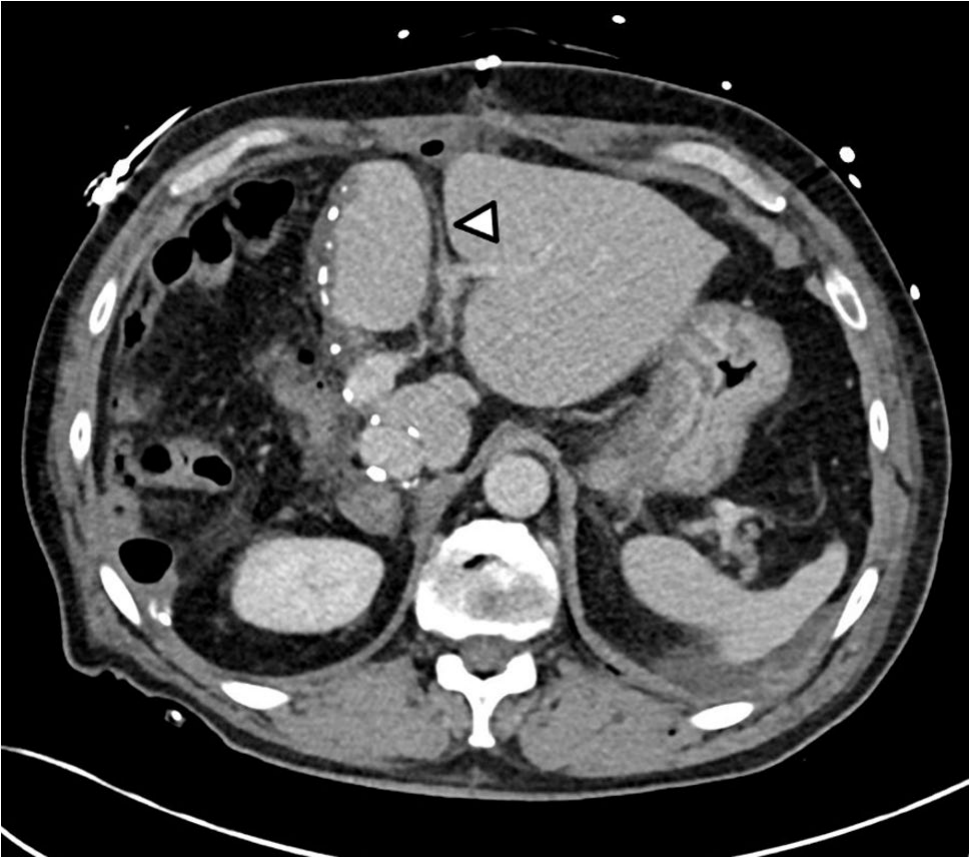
CT scan showing the postoperative recanalization of the umbilical vein. Arrow indicates the reopened umbilical vein
